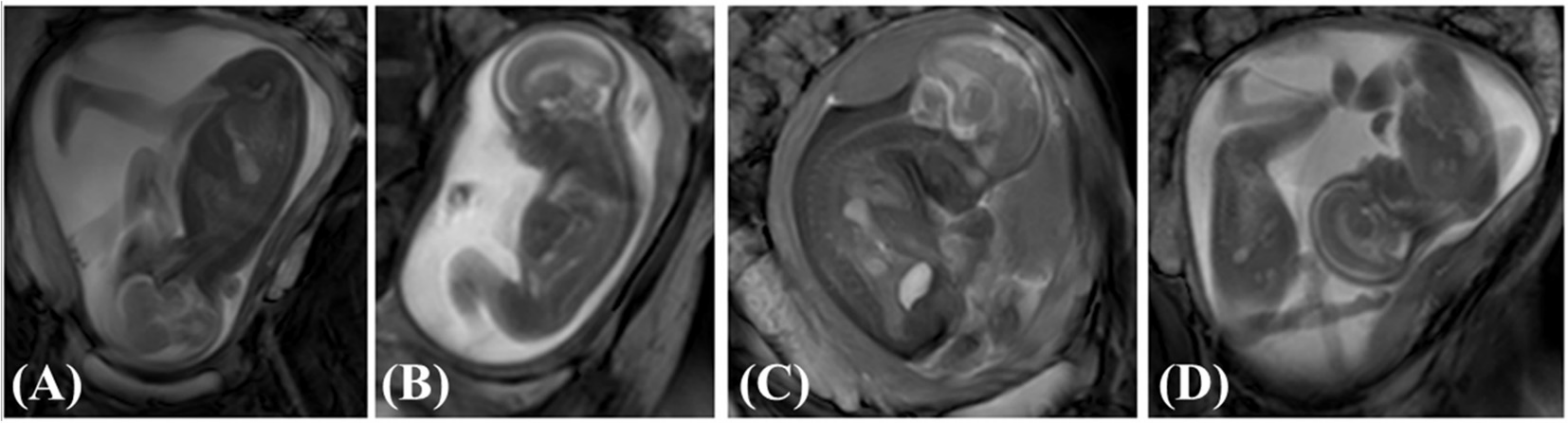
Stills from cine-MRI scans of (A) normal singleton, (B) breech position, (C) oligohydramnios and (D) twin pregnancies.
